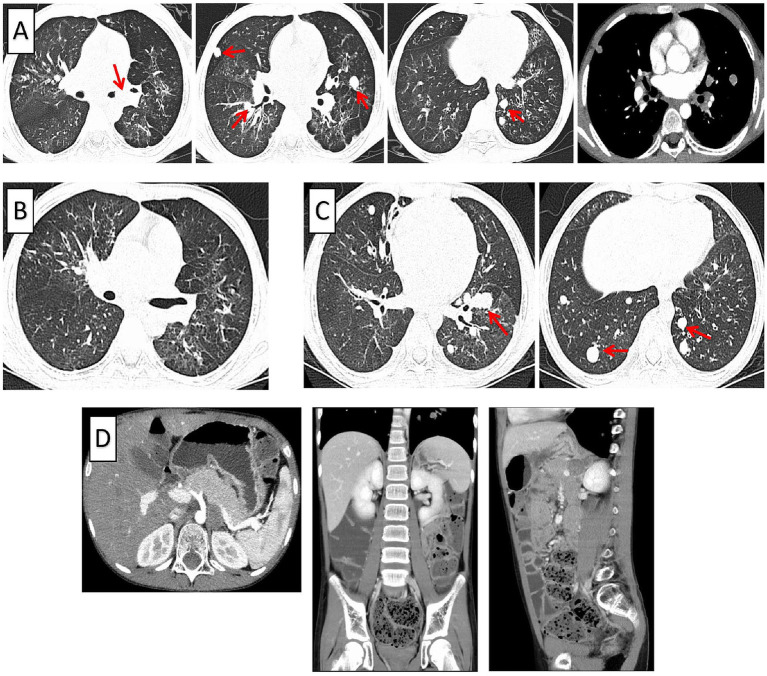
Chest computed tomography (CT) findings over time. (A) Chest CT on August 21, 2023, showing multiple nodular shadows distributed in both lungs and along some bronchi, with localized bronchiectasis in the left lower lobe. (B) Chest CT on September 13, 2023, demonstrating improved aeration of the left main bronchus following bronchoscopic resection of the endobronchial mass. (C) Chest CT on August 13, 2024, revealing an increased number and size of multiple round soft-tissue. Density nodules in the lower lobes of both lungs and bronchial involvement compared with prior imaging. (D) Abdominal plain and contrast-enhanced CT on August 15, 2024, depicting a lobulated, iso- to slightly hypodense mass in the left retroperitoneal adrenal region (approximately 2.6 cm× 1.4 cm× 2.6 cm) with poorly defined margins, an apparent capsule, and moderate enhancement with prominent capsular enhancement, indistinguishable from the left adrenal gland. Concentric soft-tissue. Density masses are also noted within the small intestine adjacent to the mid and lower poles of the left kidney, inseparable from the intestinal wall, with delayed enhancement.
